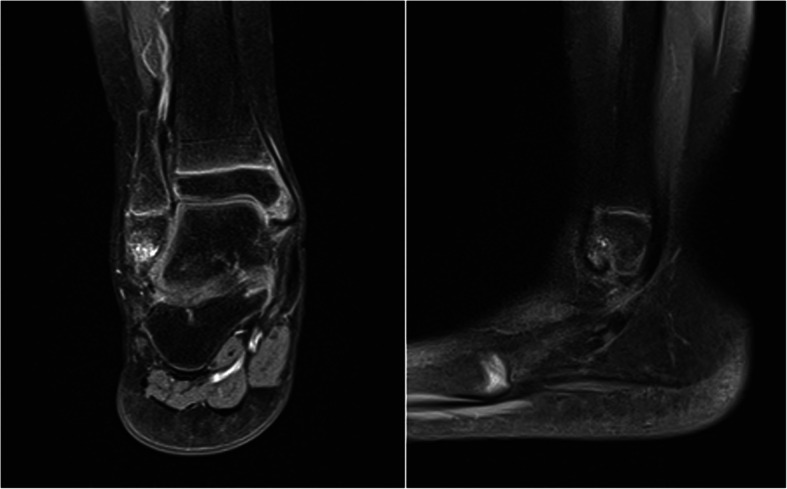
Anteroposterior and lateral MRI. The non united fragment is visible at the apex of the me the lateral malleolus, with an anomal signal and a signal suggestive of bone marrow edema on T2 images
